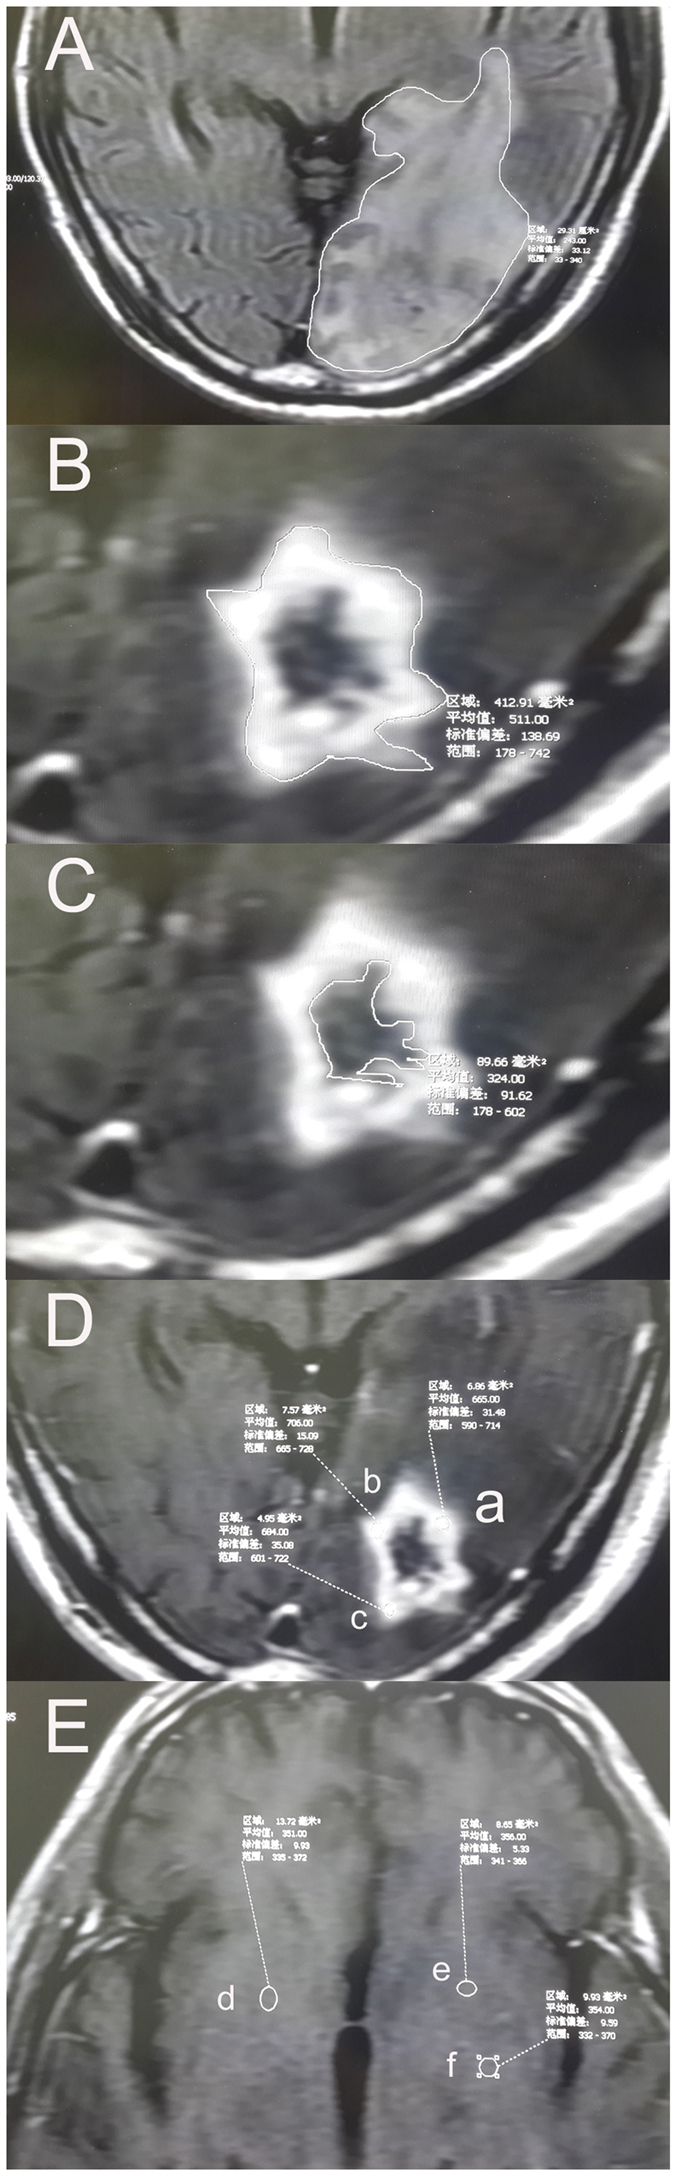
The evaluation methods of radiation brain necrosis. (A) The area of the edema at one layer of the MRI image. (B) The outer edge of radiation necrosis of the brain. (C) The inner edge of radiation necrosis of the brain. (D) Determination of MRI signal in brain necrosis area. (E) Determination of MRI signal in cerebral white matter area at the same level. The volume was calculated using the product of the area of the edema region and the slice thickness. The edema index (EI) was used for evaluation. The EI = Volume A/Volume B. The volume of cerebral necrosis was calculated using the subtraction of the inner diameter volume from the outer diameter volume of the enhancement region of cerebral necrosis(Volume B subtract Volume C). The changes in information in the region of cerebral necrosis used the measurement of the T1 strengthening phase. Three areas in the strengthening region of cerebral necrosis were selected to measure the signal values and to calculate the mean value; in addition, the value was compared with the white matter signal value of the same MRI to eliminate the influences of different strengthening degrees; this ratio was used to measure the changes in signals in the regions of cerebral necrosis before and after treatment.
